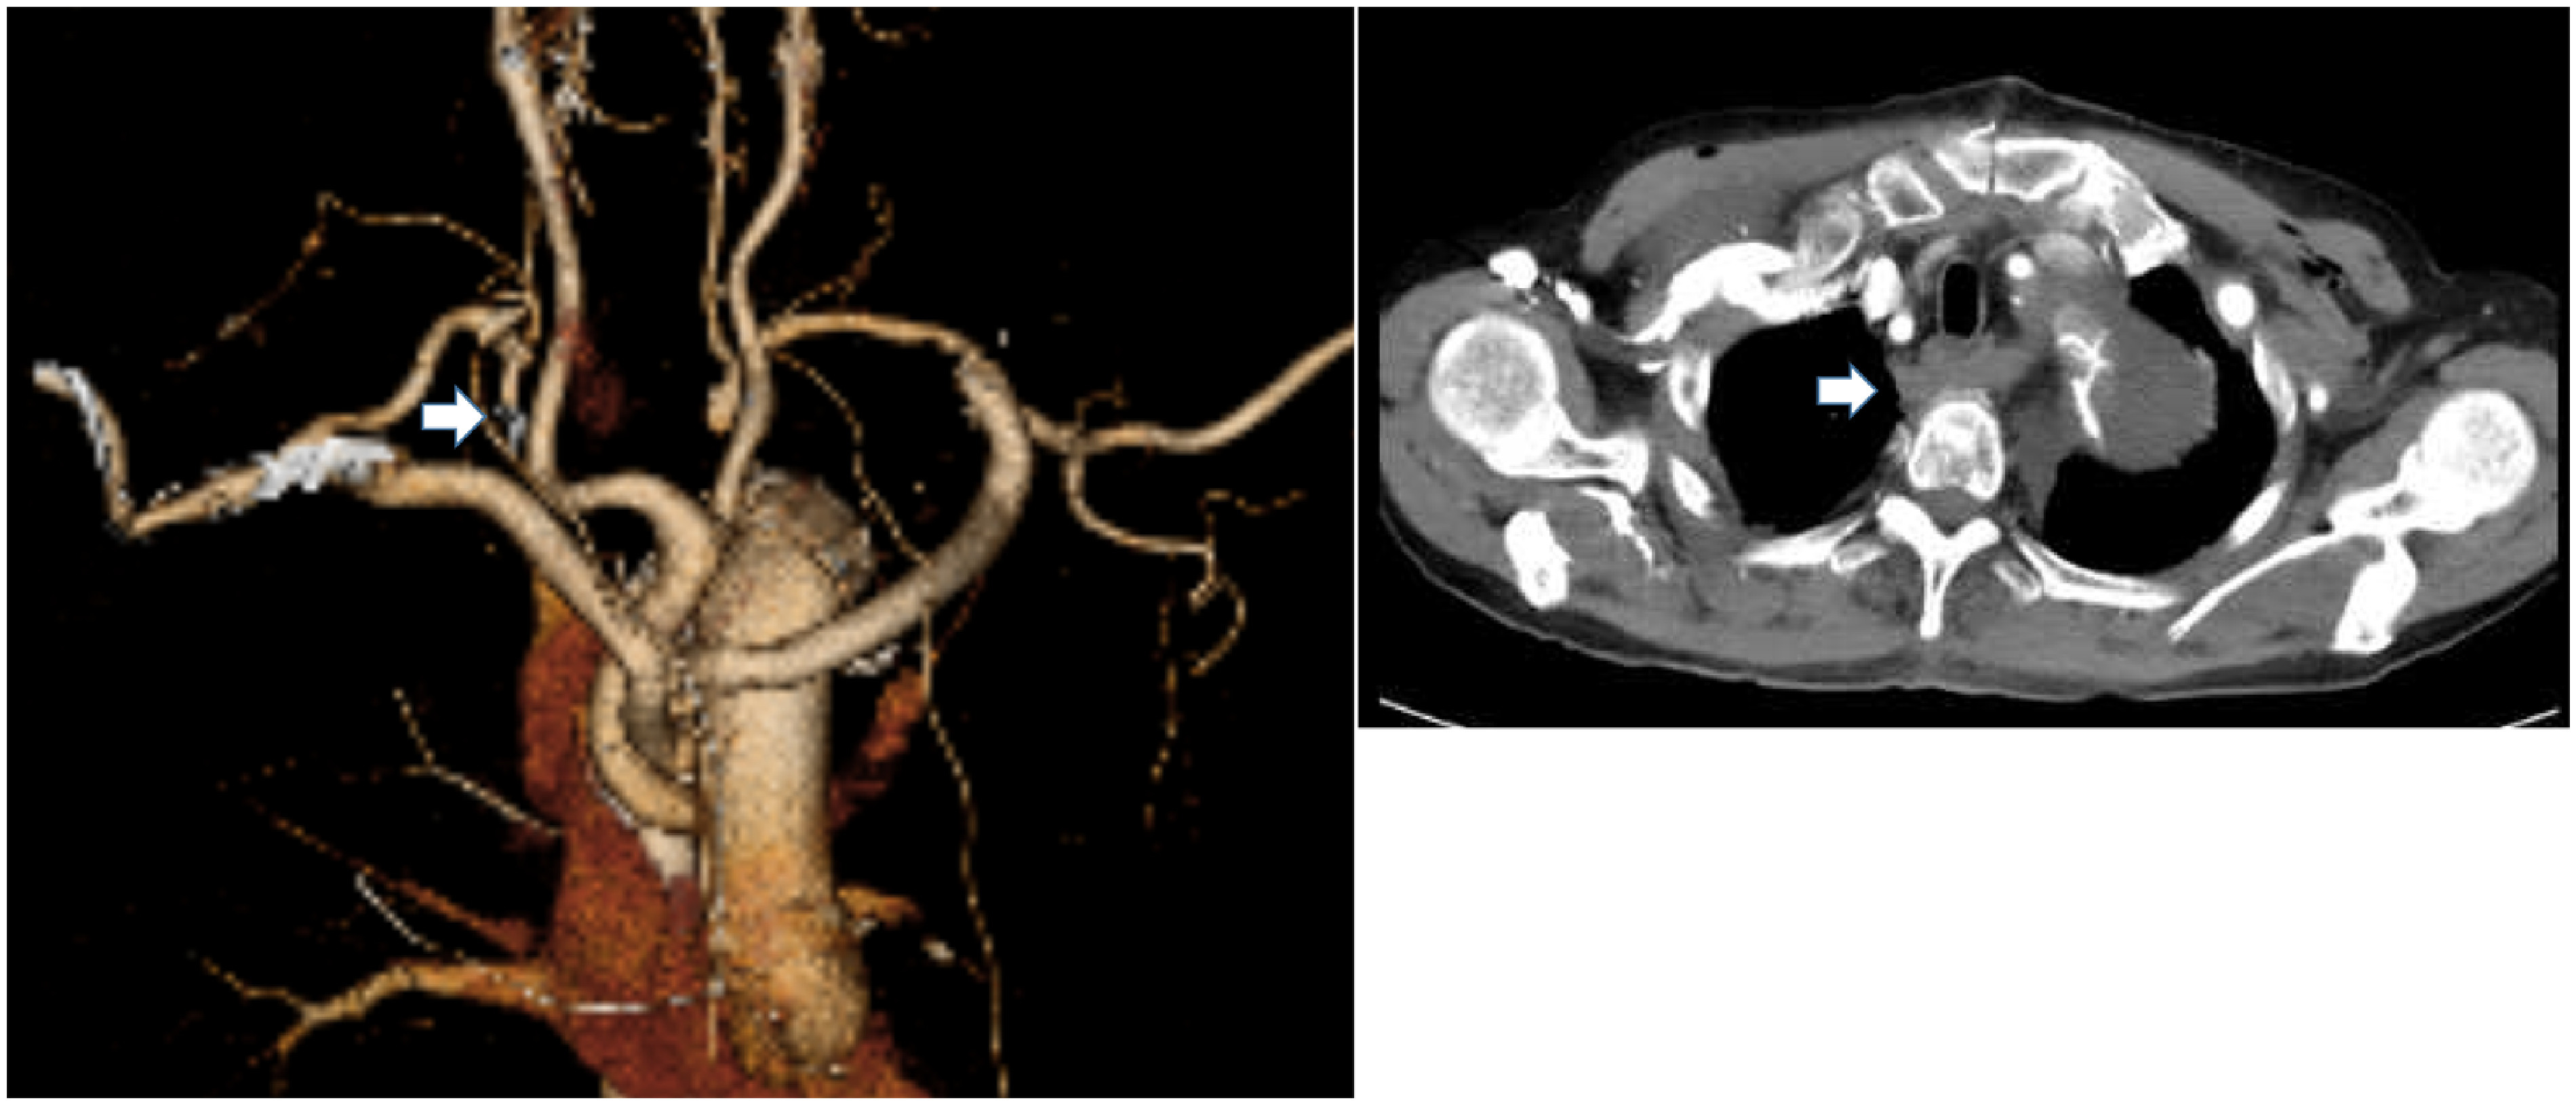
Fig. 3 Postoperative computed tomographic angiography in the frontal view presented a successful bloodstream to the bilateral carotid and subclavian arteries. Note that an aberrant right subclavian artery (arrow) was occluded by using the surgical clip (left). The axial view showed proximal aberrant right subclavian artery no longer contrasted (right).
